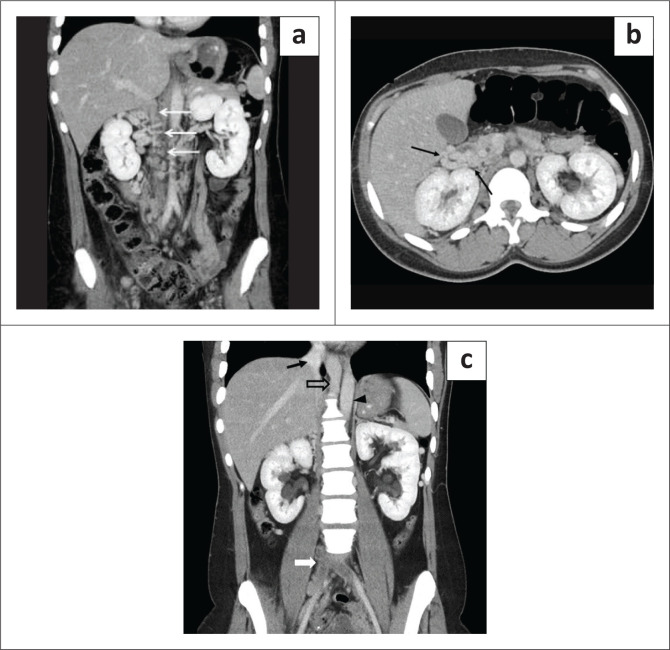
A 54-year-old female with non-specific abdominal pain. (a) Coronal contrast enhanced CT image demonstrated an absent infrarenal inferior vena cava (IVC) with multiple collaterals (thin white arrows). (b) Axial contrast enhanced CT image of the same patient shows non-visualisation of the IVC with multiple collaterals (thin black arrows). (c) Bilateral common iliac veins fuse (thick white arrow) but do not continue as the IVC, draining via multiple collaterals into the azygos vein (open black arrow) seen to the right of aorta (black arrowhead). The suprahepatic IVC (black solid arrow) is present, draining into the right atrium.
